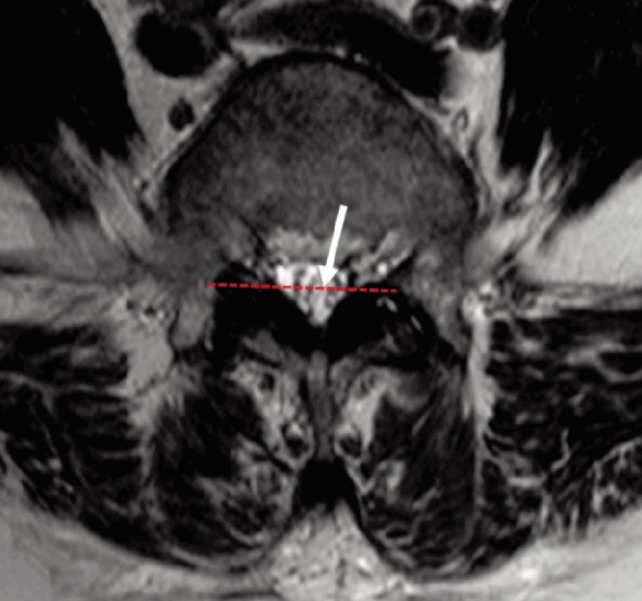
MRI T2 image of a patient with positive nerve root sedimentation sign. A positive sign was defined when most of the nerve roots (arrow) in the dura sac was located in the area above an imaginary line (dotted line) dividing the spinal canal into two halves.
